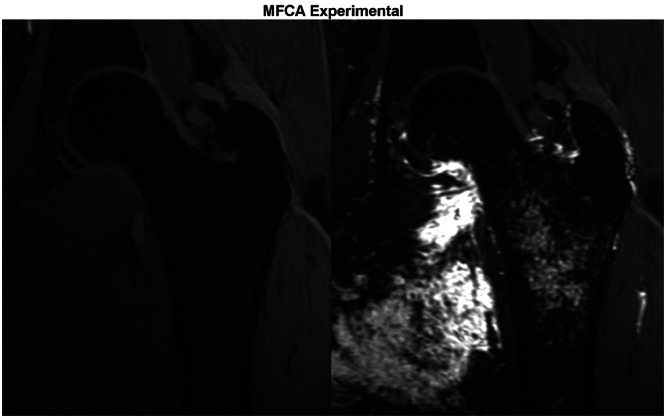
MRI, including pre‐contrast (left image) and post‐contrast (right image), demonstrating arterial contributions from the MFCA. MFCA, medial femoral circumflex artery; MRI, magnetic resonance imaging.
